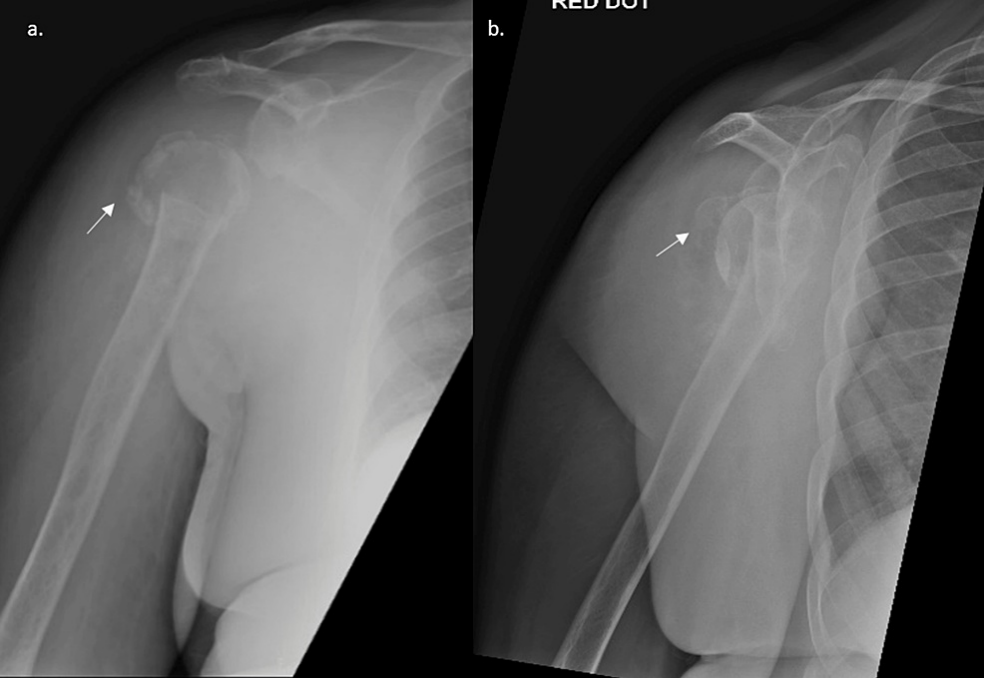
Pre-operative radiographs a. AP b. PA radiograph of the right shoulder depicting degenerative changes to the humeral head consistent with Charcot arthropathy (white arrows).
